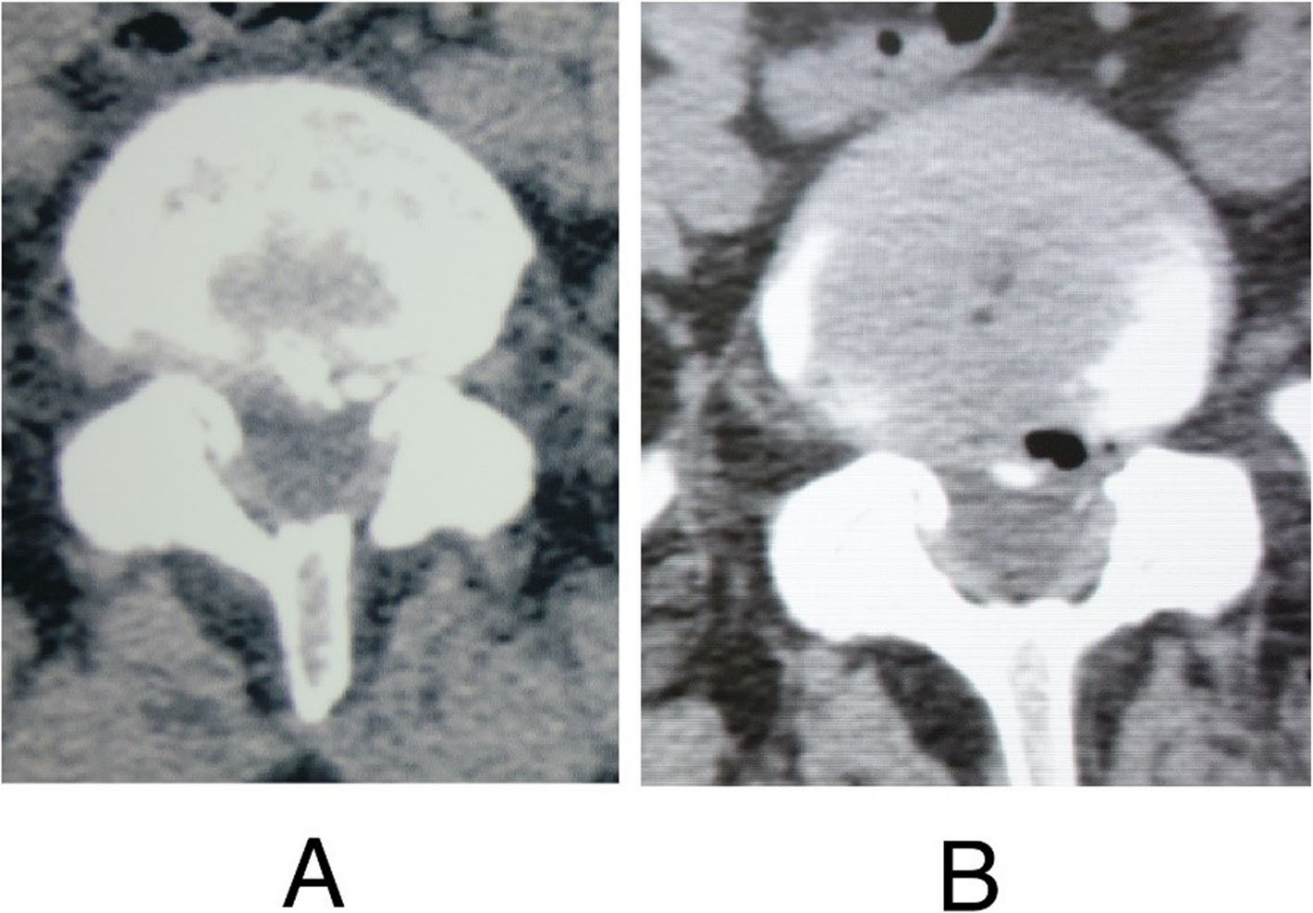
Preoperative and postoperative CT scan images of a 46-year-old female patient who had radiating leg pain for one month and received PTES. .a Preoperative CT scan image showed calcified herniation at L5/S1 level. b Postoperative CT scan image confirmed the removal of the calcification
